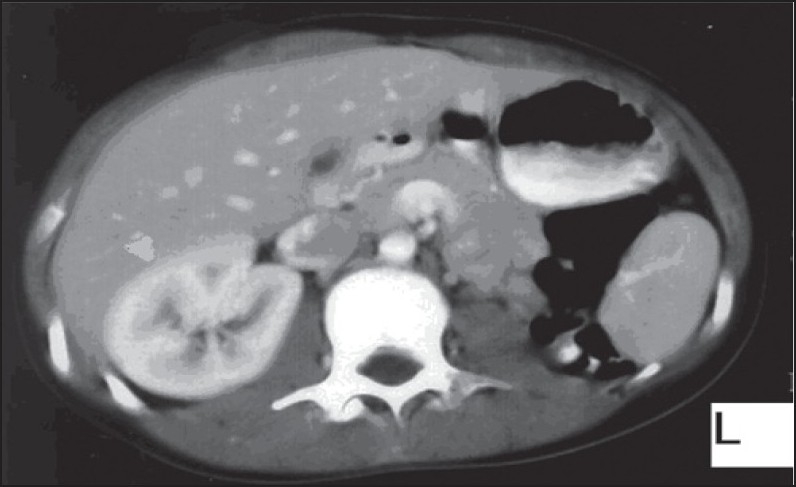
Abdominal CT scan.What's your diagnosis?
